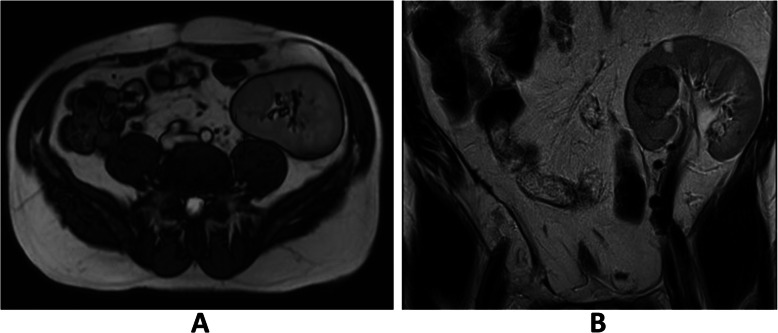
a. Magnetic resonance imaging of the abdomen: tumour in the cortex of the transplanted kidney. Turbo spin echo (TSE), fat suppression sequence, transverse plane. b. Magnetic resonance imaging of the abdomen: tumour in the cortex of transplanted kidney. Cortical cyst in the upper pole of the transplanted kidney. Turbo spin echo (TSE), fat suppression sequence, frontal plane
